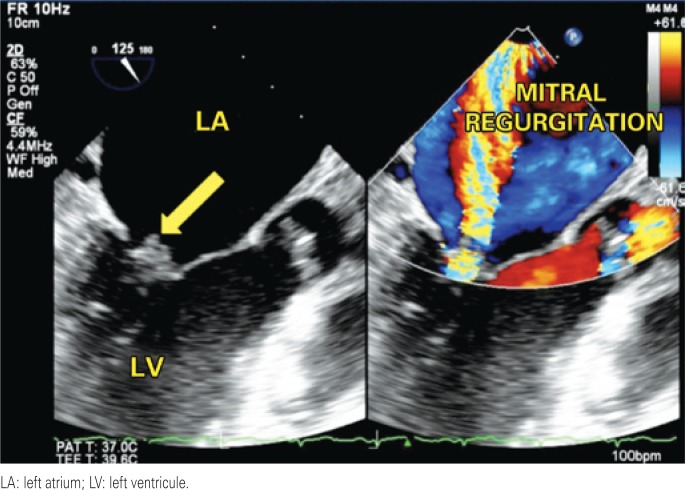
Two-dimensional transthoracic echocardiogram showing vegetation (arrow) and mitral regurgitation
